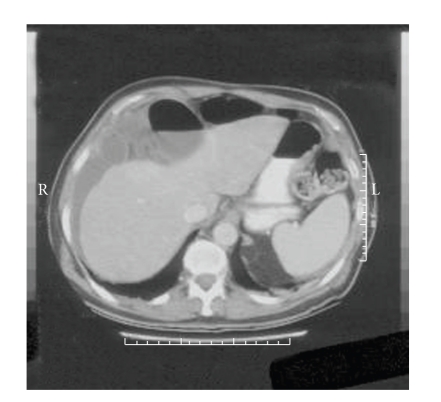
Representative section from the patient's abdominal CT scan. Dilated, fluid-filled loops of small and large bowel are noted anterior and superior to the liver, along with free fluid.
